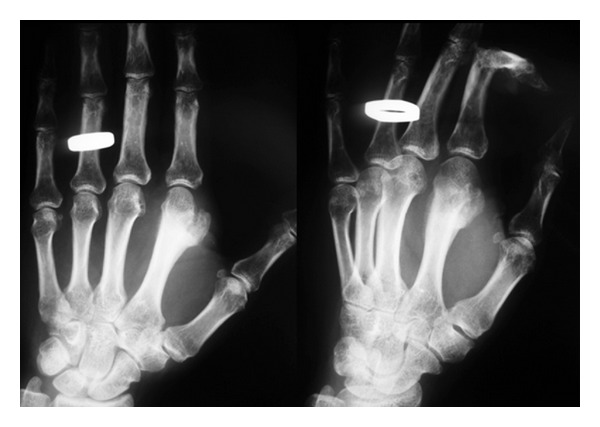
Case  2: an AP and oblique radiographs of the hand demonstrating a somewhat pedunculated mass arising from the lateral surface of the second distal metacarpal and resembling a focus of mature ossification with distinct medullary and cortical components.
